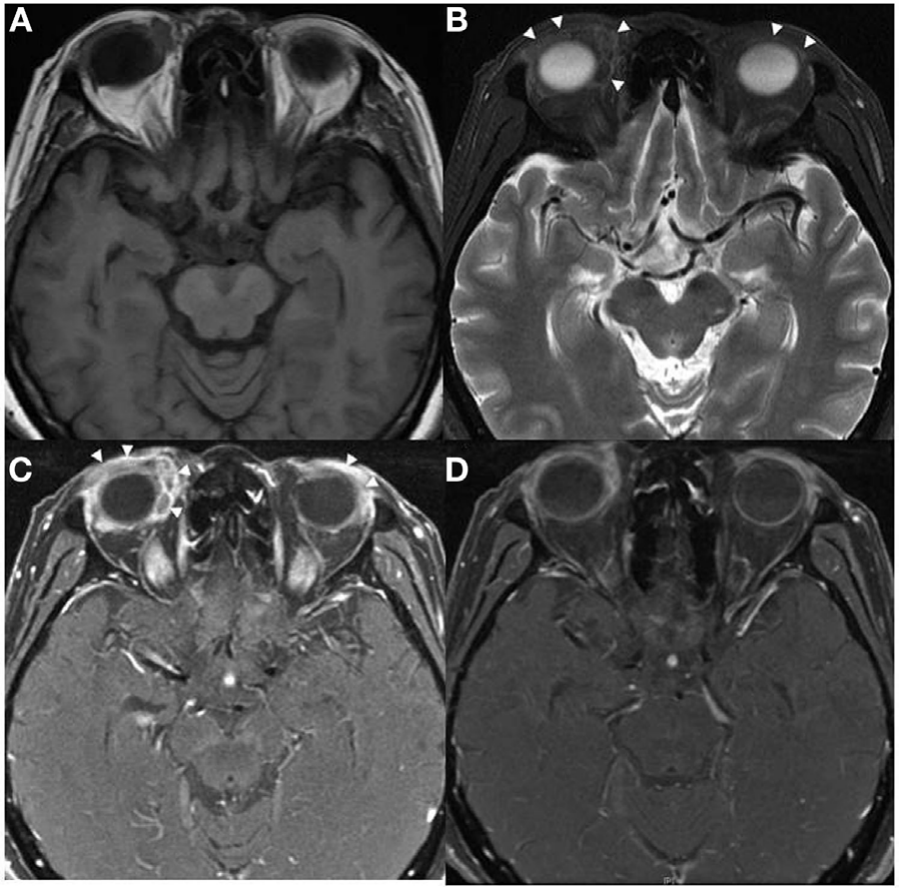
Axial MRI of patient 1 showed predominately extraconal infiltrating soft tissue lesions in both orbits (arrowheads) with significant preseptal component, they were hypointense on T1W (A) and T2W (B) images with heterogeneous contrast enhancement (C,D).
